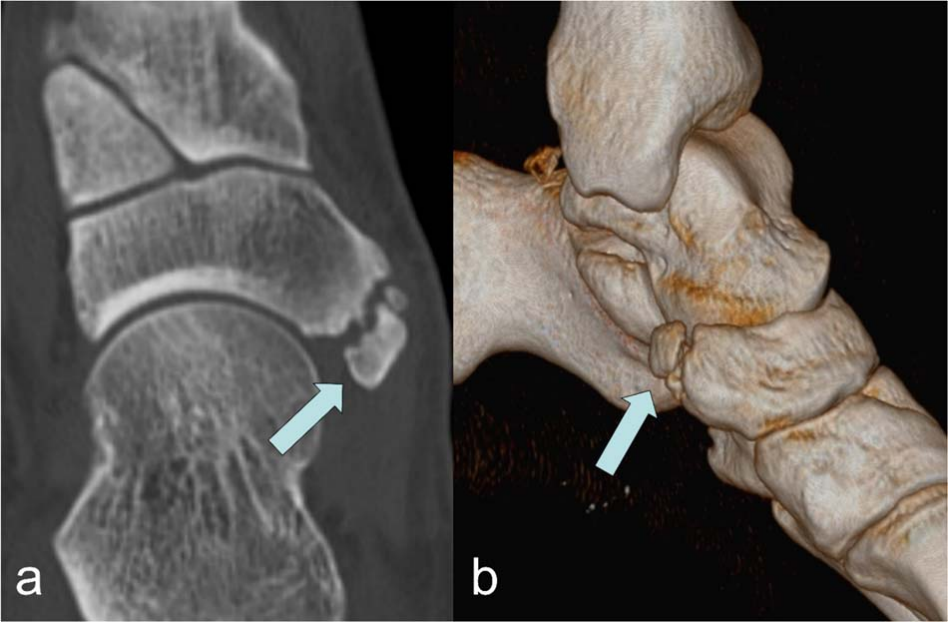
Image of the Os tibiale externum Type II in the coronal reformatted CT scan (a) and in the 3D representation (b); the ossicle is marked with an arrow.
